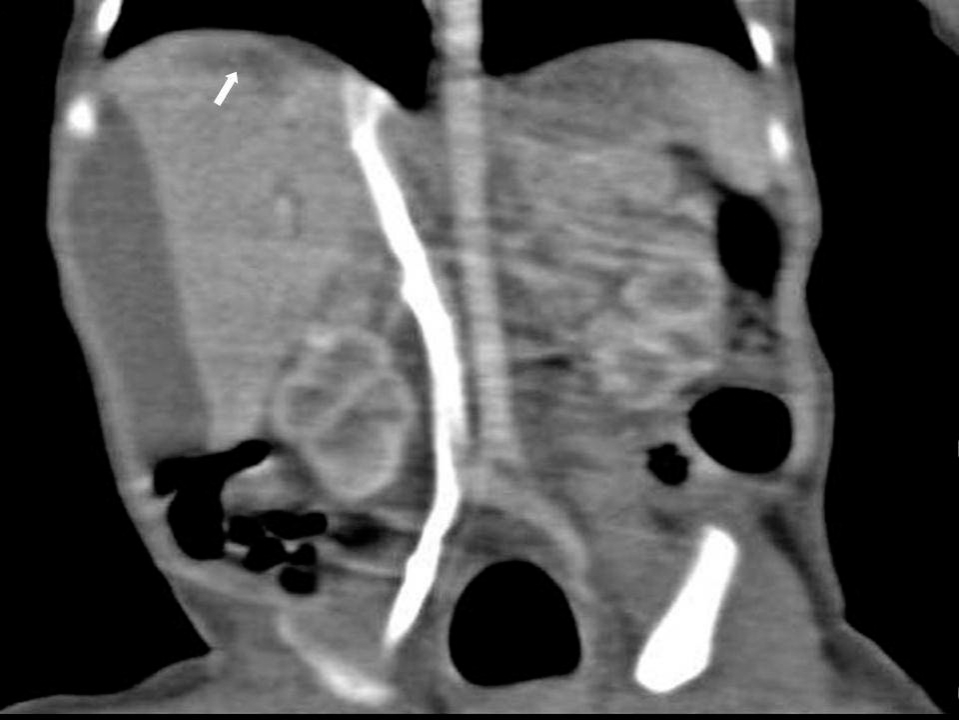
A multi-planar reformatted coronal CT scan shows a partial linear low-density lesion in the dome of the liver presumed to be parenchymal laceration (arrow). An non-enhancing cystic mass is seen in the lateral margin of the liver with hepatic compression.
